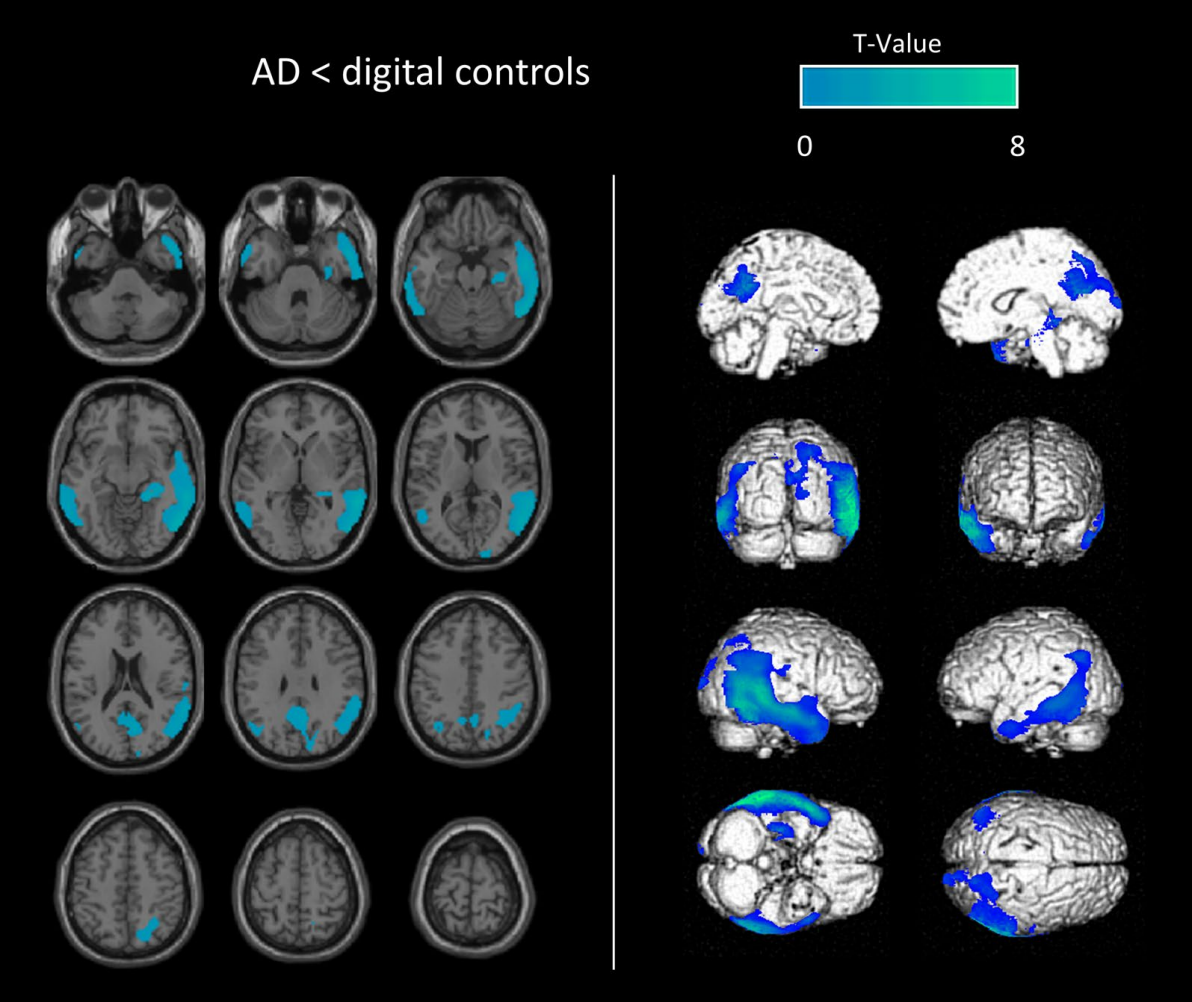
Example of SPM-T map images used for the visual analysis (semi-quantitative analysis to digital controls) of a patient with AD (79-year-old man with MMSE score of 10). SPM-T maps are projected onto two-dimensional slices of T1-weighted MRI (from the base to the top of the skull, left panel) and 3D-rendered volumes (right panel)
